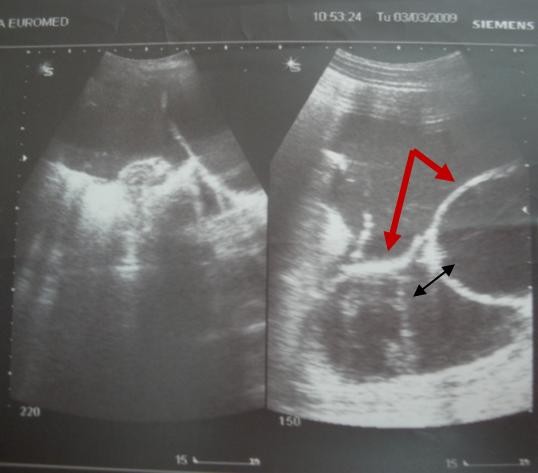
Ultrasound evaluation typically shows a thin-walled cystic mass (thick arrow), with fine internal septa (thin arrow).
